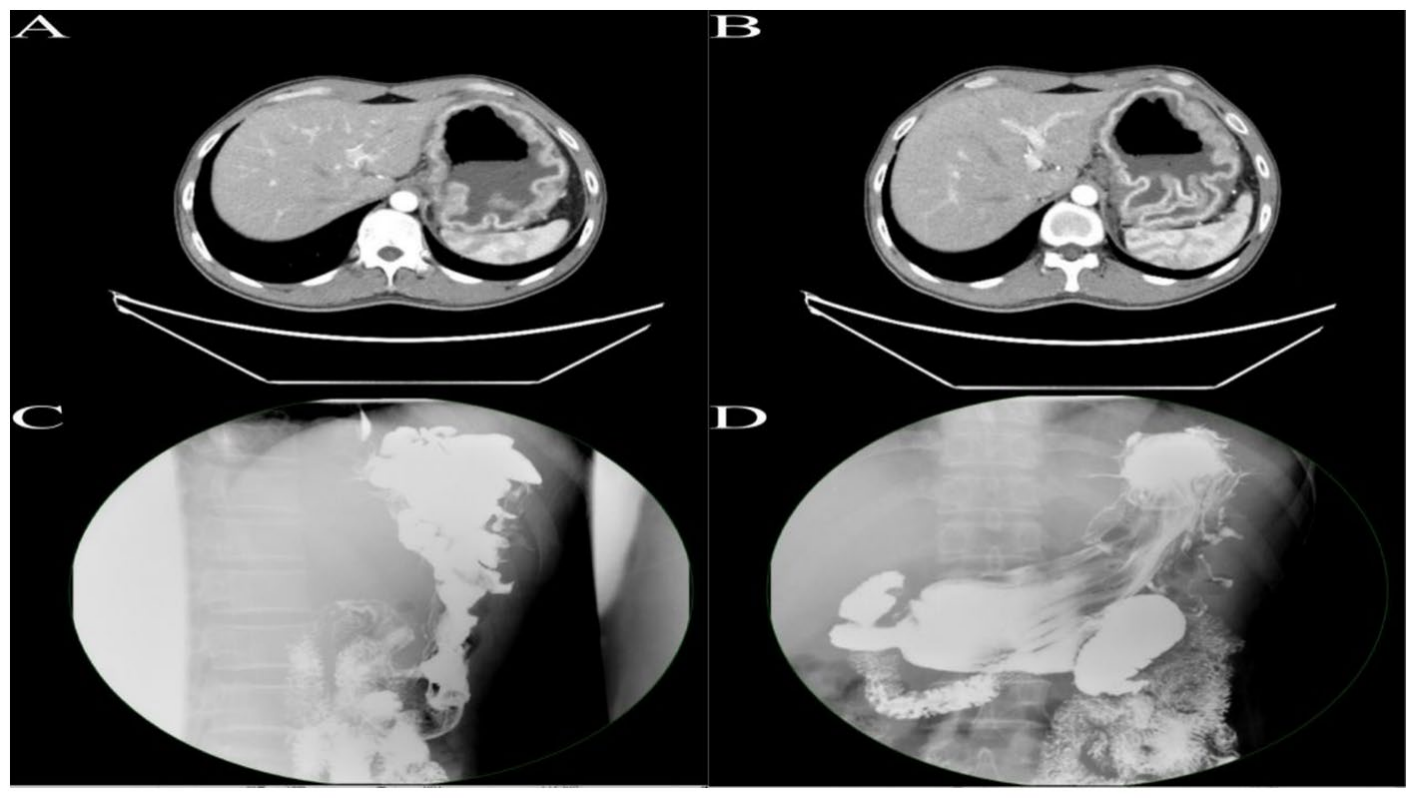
(A,B) Enhanced CT of the upper abdomen reveals gastric distension with significant mucosal thickening exhibiting a corrugated appearance, with pronounced enhancement on contrast imaging. (C,D) Upper gastrointestinal series demonstrates marked hypertrophy of the mucosal folds in the fundus and body of the stomach, presenting with polypoid and convoluted characteristics, with some interfold spaces appearing irregular.
